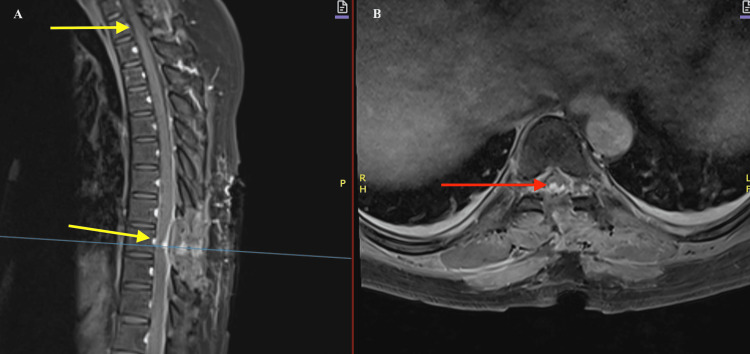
Postoperative day 0 contrast-enhanced MRI of the thoracic spine Sagittal (A) and axial (B) contrast-enhanced MRI images show a small residual focal area of enhancement within the spinal cord, to the right of midline and extending along the posterior aspect of the cord (red arrow), representing residual tumor versus postoperative changes. Residual spinal cord edema extending from the inferior endplate of T9 to the mid-T1 vertebral body (yellow arrows) is unchanged compared with Figure 1.
